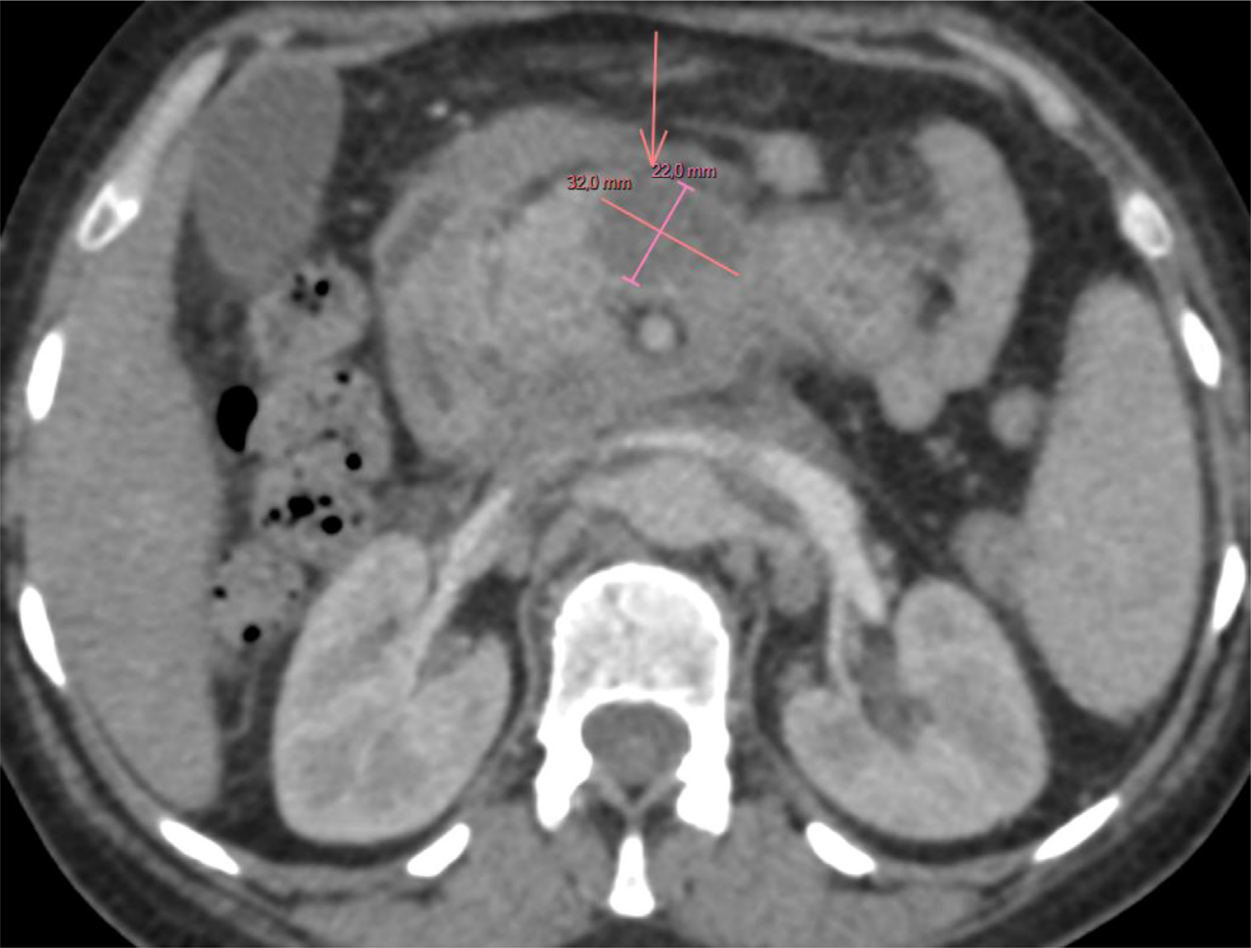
CT scan of the third case, transverse section, demonstrating hypertrophy of the pancreatic gland with a cystic area.
